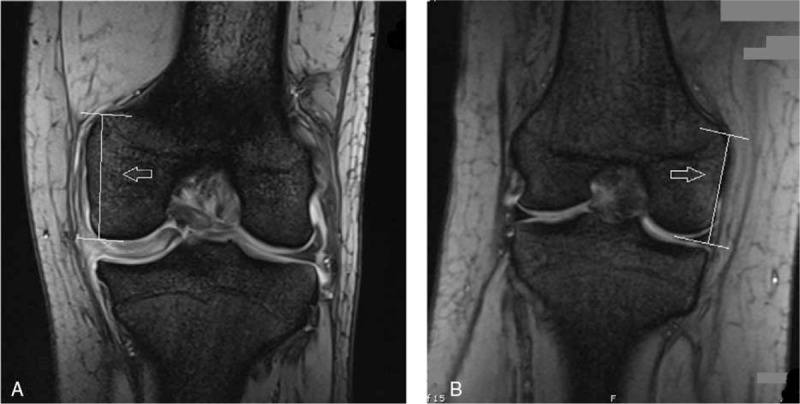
MRI image of the patient (A, left knee) and a normal knee (B, right knee) in the coronal plane. Relative to the height of the lateral femoral condyle, the patient's femoral medial condyle is shorter than would be expected in a normal knee (indicated by the white arrow). MRI = magnetic resonance imaging.
